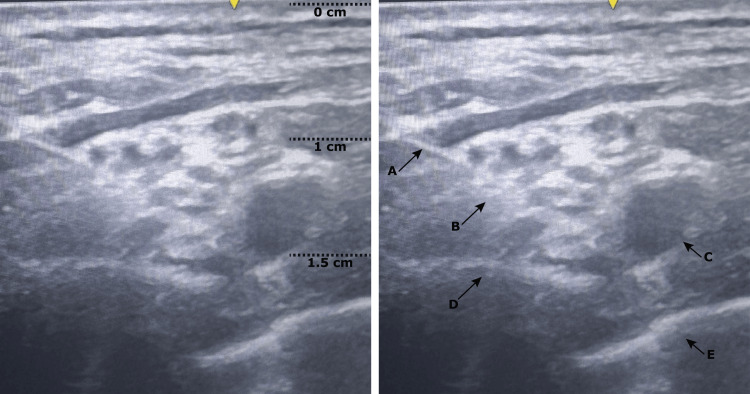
Ultrasound-guided supraclavicular brachial plexus block Transverse ultrasound image of the right supraclavicular region. The needle and relevant anatomical structures are identified by arrows and letters. The needle (A) is visualized advancing toward the brachial plexus (B), at an approximate depth of 1.5 cm, located lateral to the subclavian artery (C). The first rib (D) appears as a hyperechoic structure with posterior acoustic shadowing, and the pleura (E) is identified inferiorly. This visualization optimizes the needle trajectory while maintaining a safe distance from vascular and pleural structures
